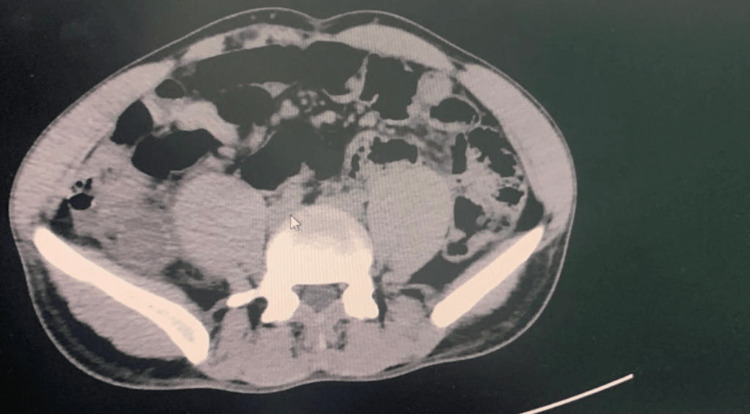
Contrast-enhanced computed tomography of the abdomen showing a tubular mass in the appendiceal area.
